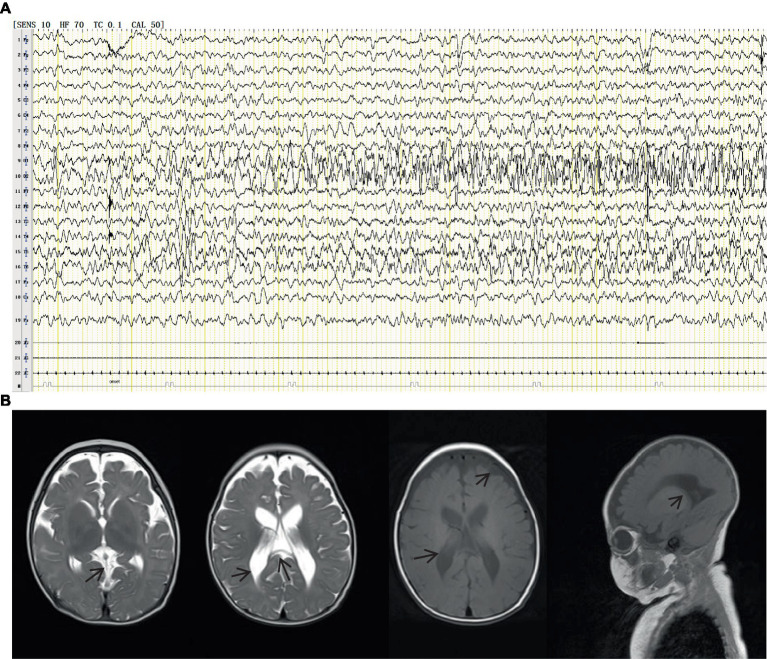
VEEG during the seizures showed that spike wave rhythms of medium to high amplitude first occurred at the electrodes in the occipital and posterior temporal regions, which later affected other regions (A). Cranial magnetic resonance imaging (MRI) showed enlarged bilateral lateral ventricles and Vergae ventricle, the corpus callosum was thinned and the anterior temporal extracerebral space was widened bilaterally, with the bilateral lateral ventricles obviously deformed (B).
